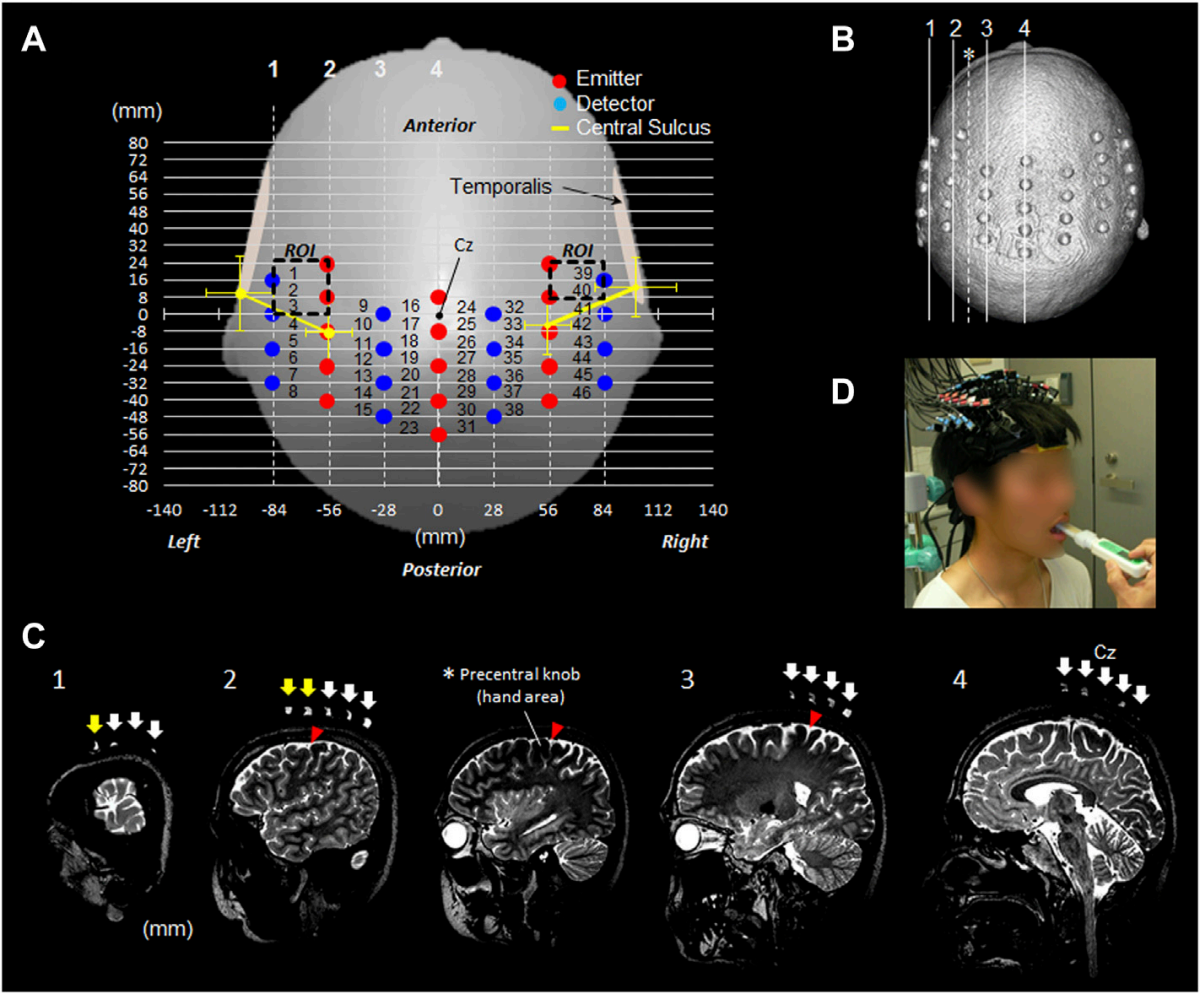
fNIRS measurement settings. (A) fNIRS 46-channel arrangement and the estimated bilateral OMC. (B) Head image reconstructed from 3D T2-weighted MRI taken with a registration marker, which indicates the probe position. The four lines from 1 to 4 correspond to the positions in the image in Figure (C). (C) Sagittal images 1–4 along the registration marker are shown. The sagittal image presented with an asterisk indicates a slice passing through the precentral knob between rows 2 and 3. Among the arrows indicating the probe position, the yellow arrow of slices 1 and 2 indicates the position of the pair probe sandwiching the part corresponding to the OMC. (D) Participant wearing a probe during experimental tasks. OMC: oral motor cortex. ROI: region of interest. Cz: location of vertex on the scalp.
